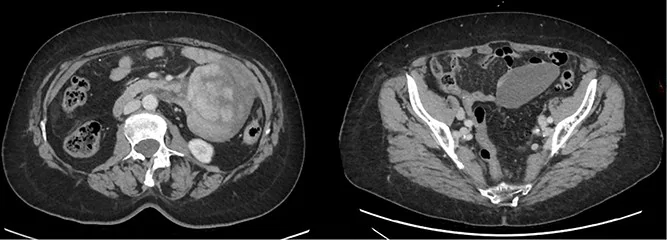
Axial CT-scan: the retroperitoneal liposarcoma on the left and the mesenteric liposarcoma on the right.
